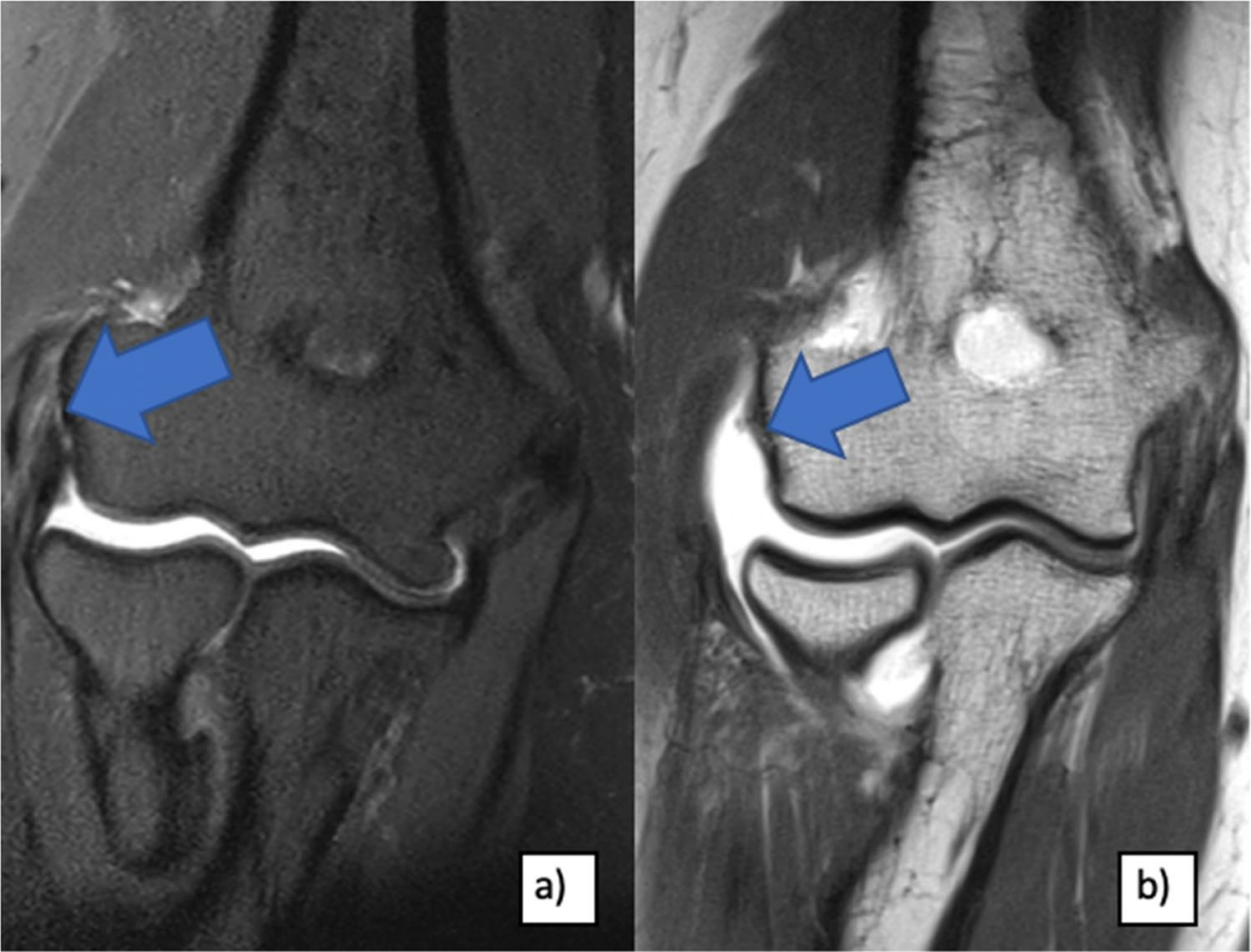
MRI of patients with atraumatic LUCL insufficiency (right elbow): a partial lesion of LUCL and extensor tendons (blue arrows) after multiple steroid injections and b complete LUCL and extensor tendon avulsion (blue arrow) following Hohmanns’ surgery
